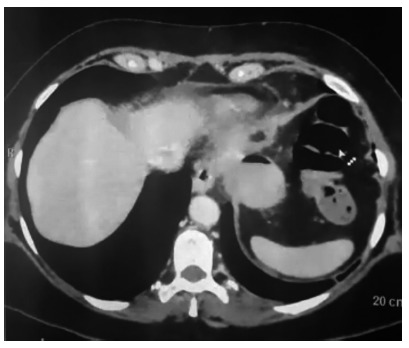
CT demonstrating and adequate Nissen wrap with no complications and a normal sleeve size.
